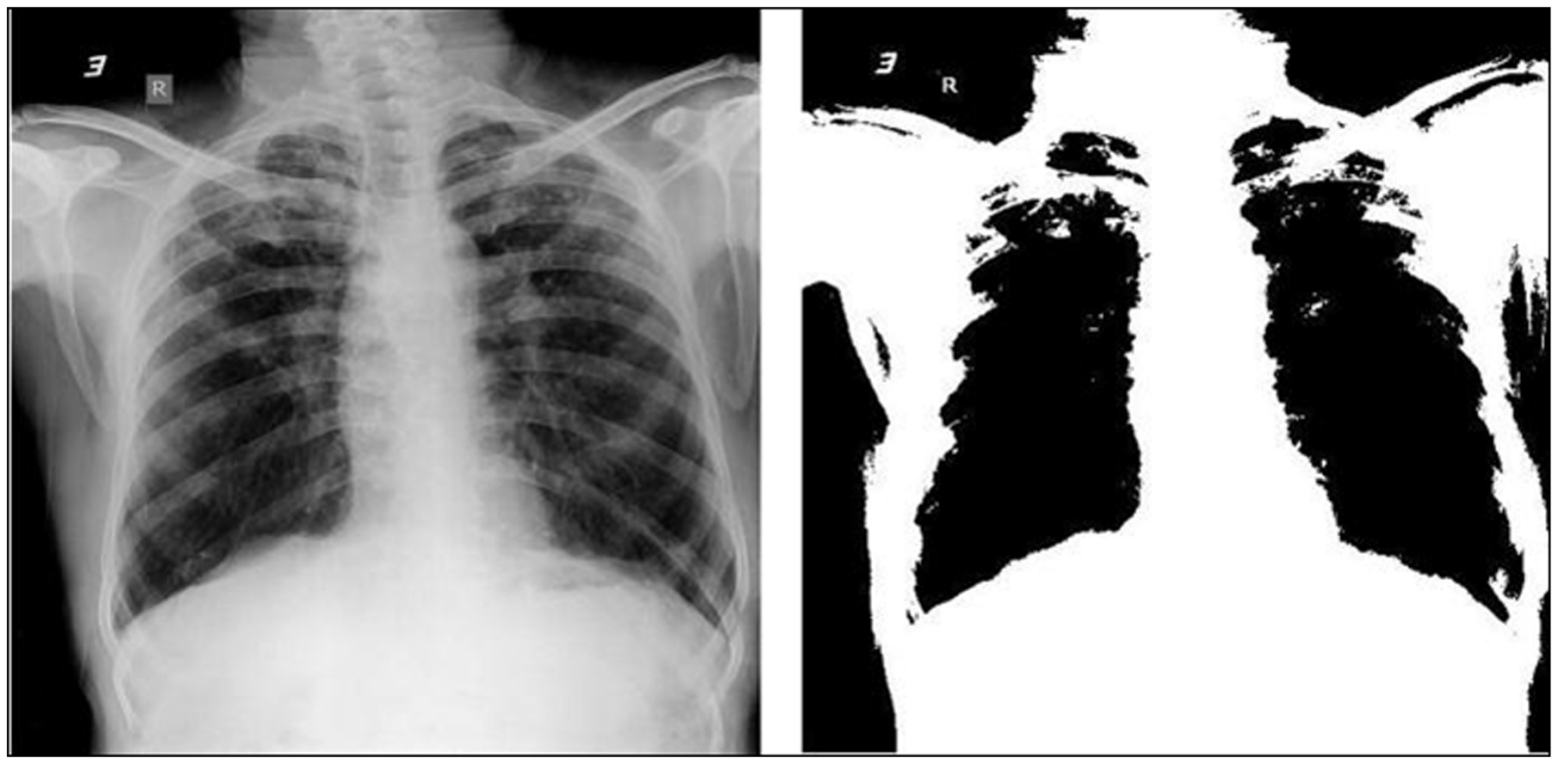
X-ray image before and after Euler-based segmentation. The Euler-based segmented image provides initial contour for Chan-Vese segmentation.
